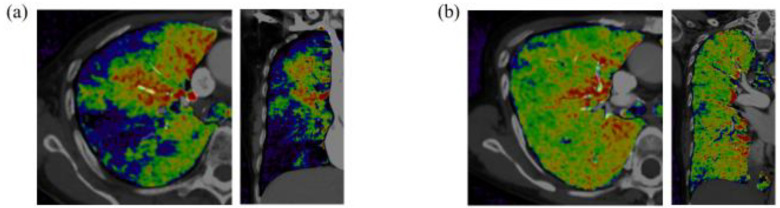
Representative horizontal section and coronal section of DE-CT images in a patient with CTEPH with poor subpleural perfusion (a) and PAH (b). Black-blue color-coded image represents poor lung perfusion, and red-yellow color-coded image represents well lung perfusion.
